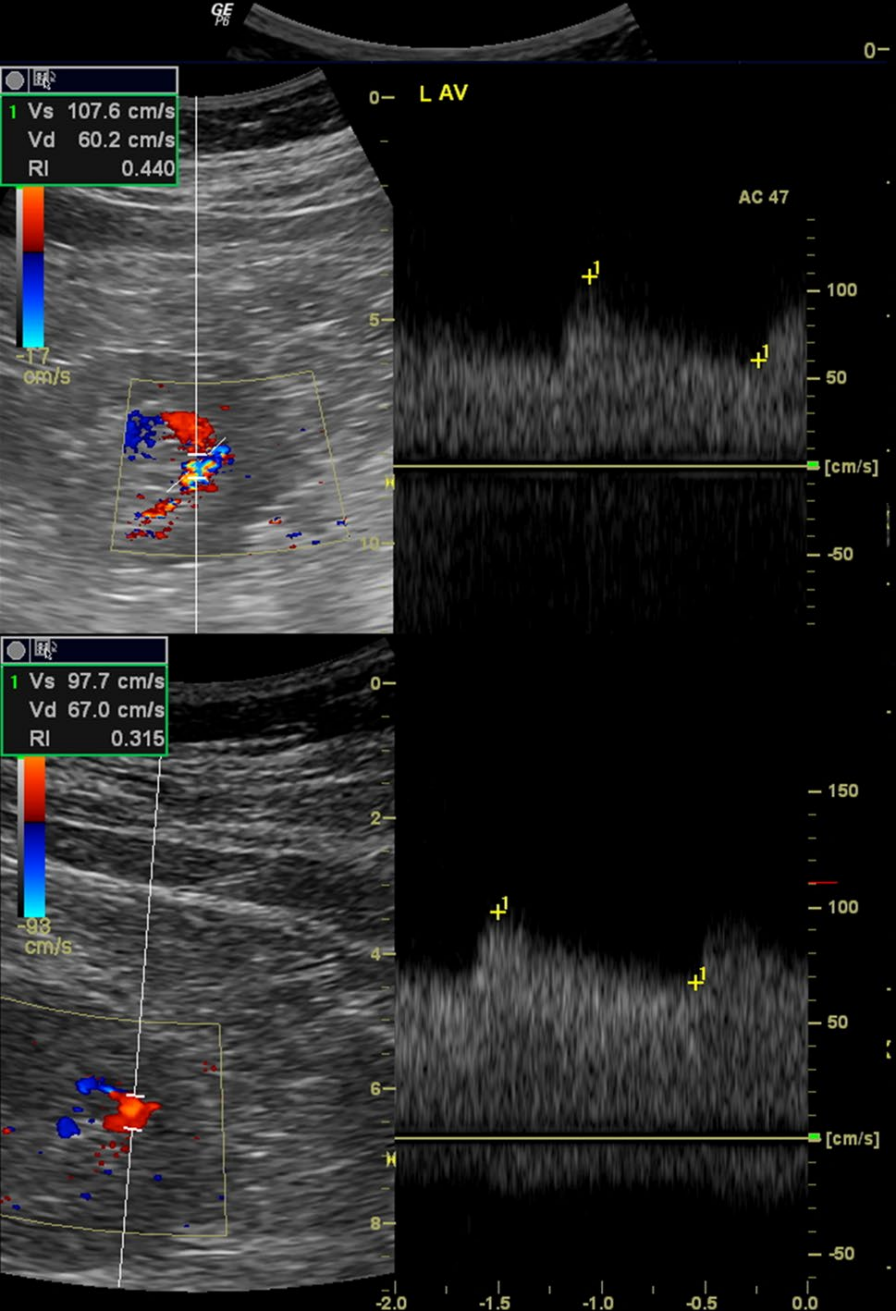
Doppler hemodynamic assessment of renal biopsy-related arteriovenous fistulas
